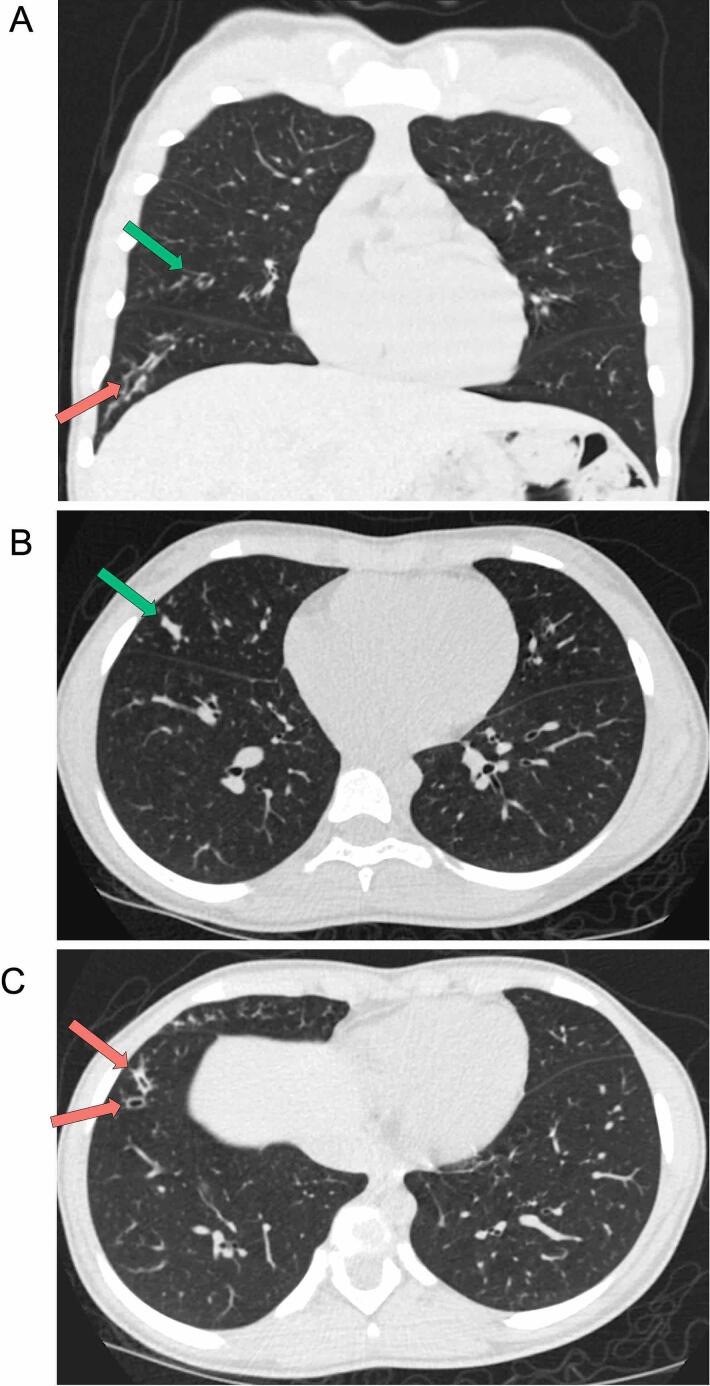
Chest CT at 11.8y identifies pathological respiratory features. Mild bronchiectasis (right lower lobe, red arrows) and mucus plugging (right middle lobe, green arrows) are demonstrated on coronal (A) and axial (B-C) views. For interpretation of the references to color in this figure legend, the reader is referred to the web version of this article.
